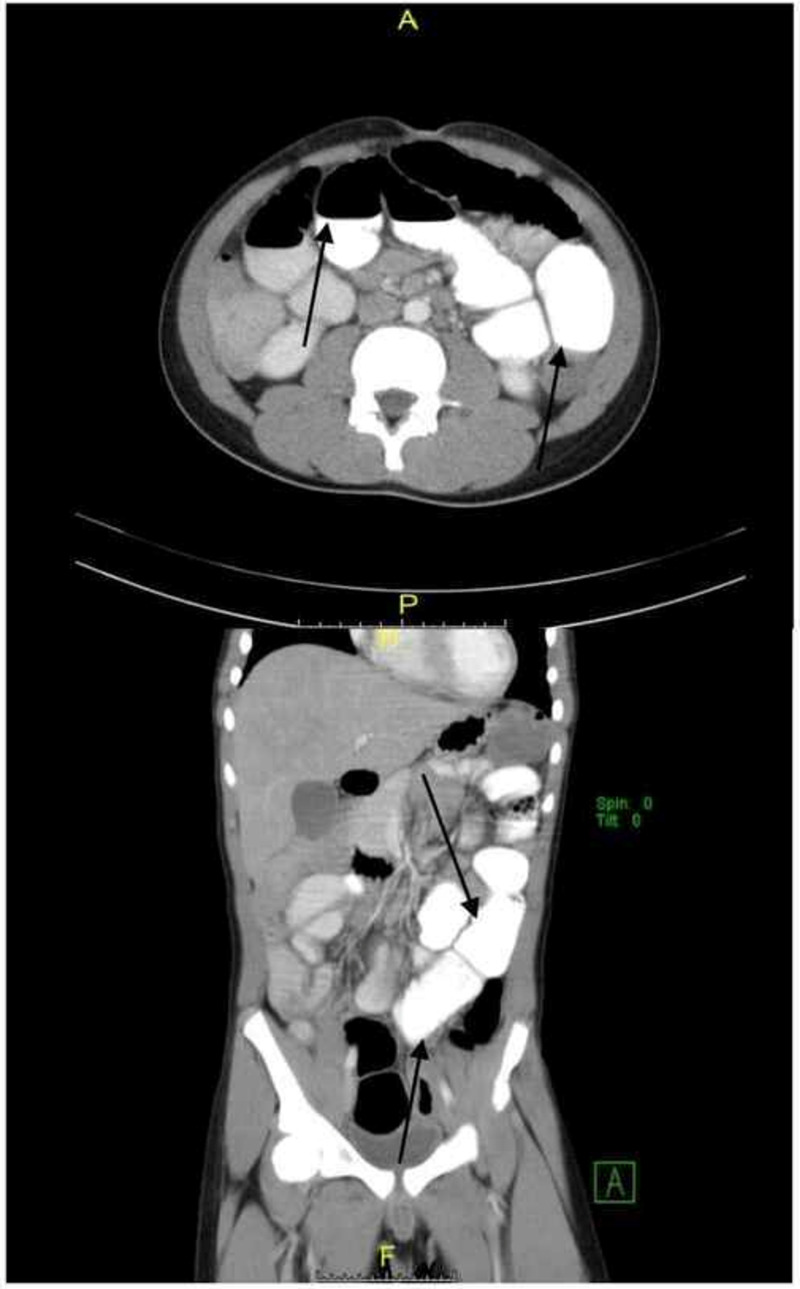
CT abdomen pelvis with contrast The image shows mild dilation of the entirety of the jejunum and ileum without a transition point and which may represent an ileus. Contrast is present throughout the small bowel, as well as in the stomach, and has reached the colon indicating there is likely no mechanical obstruction (black arrows). Continued radiographic follow-up was recommended CT: computed tomography
